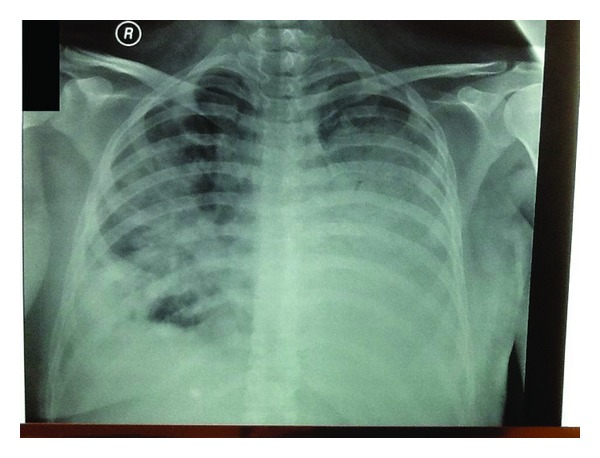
Chest radiograph of day 2 showing diffuse alveolar opacities extending to the left upper lung fields, but in the right middle and lower lobe.
